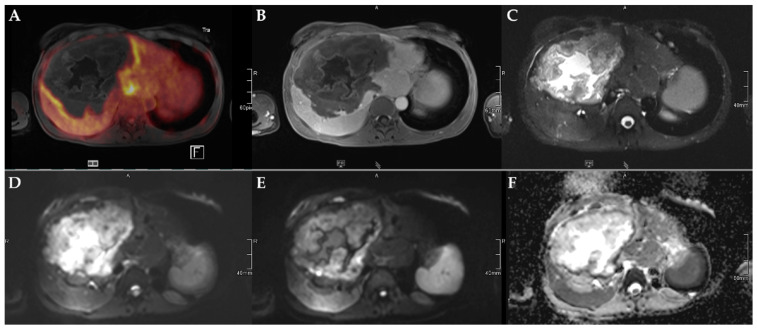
45-year-old female patient with AE and involvement of liver segments IVa–VII and Kodama Type 3 lesion. Increased FDG uptake is observed at the lesion rim (A) with increased uptake of the contrast agent (B). High signal intensity is present in the central cystic parts in T2w imaging with fat suppression (C). Diffusion imaging demonstrates a high signal at a low b-value (b50) in the central parts of the lesion (D), a low signal at a high b-value (b800) (E), and high ADC values (F), indicative of no diffusion restriction.
